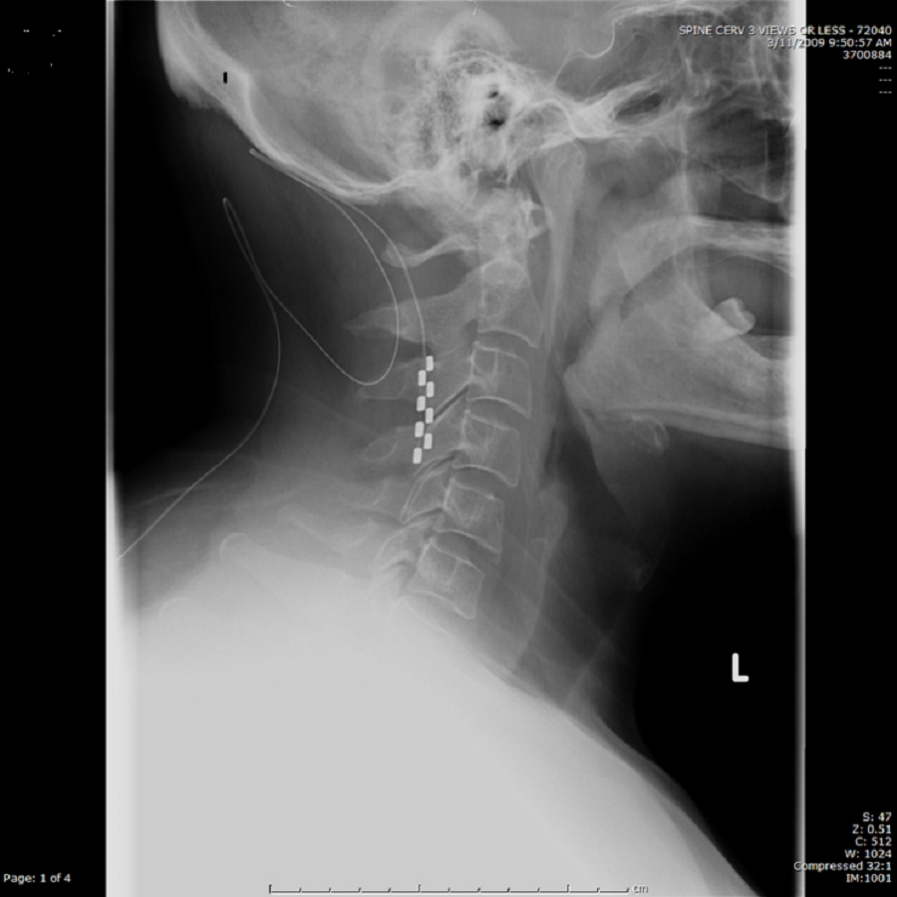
Lateral Cervical Spine X-Ray Caudal migration of dorsal column stimulator paddle lead to C3-C4
